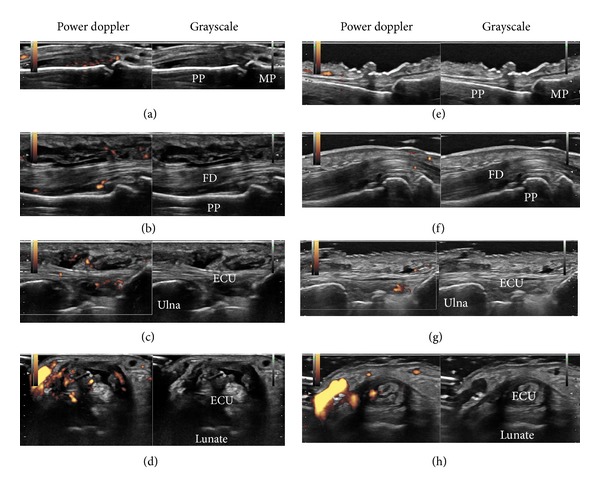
Ultrasound images of hands in Case 3 before and after treatment. Shown are the representative ultrasound images of the left hand before treatment (a–d) and after 24 weeks of abatacept treatment (e–h). Ultrasound images before treatment reveal moderate synovial hypertrophy with moderate Doppler signals in the 3rd proximal interphalangeal joint (a) and severe synovial hypertrophy with moderate Doppler signals in the flexor tendon of the 3rd finger (b) and extensor carpi ulnaris longitudinal view (c) (see also webvideo 1) and transverse view (d) (see also webvideo 2). Corresponding ultrasound images after abatacept treatment demonstrate marked improvement (e–h). PP: proximal phalanx; MP: middle phalanx; FD: flexor digitorum; ECU: extensor carpi ulnaris.
